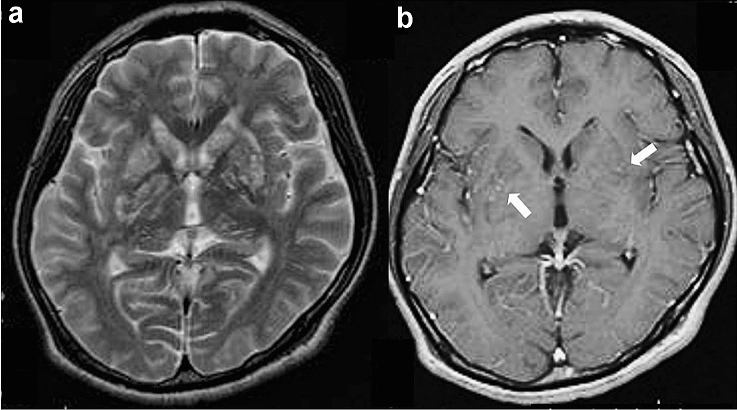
Dilated periventricular space in a patient with HIV-related CNS Cryptococcosis. T2-weighted image (a) shows clustered innumerable hyperintense cysts, characteristic of cryptococcal gelatinous pseudocysts, bilaterally in the lentiform nuclei and head of the caudate nucleus and thalamus. Some of them show contrast enhancement on contrast-enhanced T1-weighted image (b, arrows)
